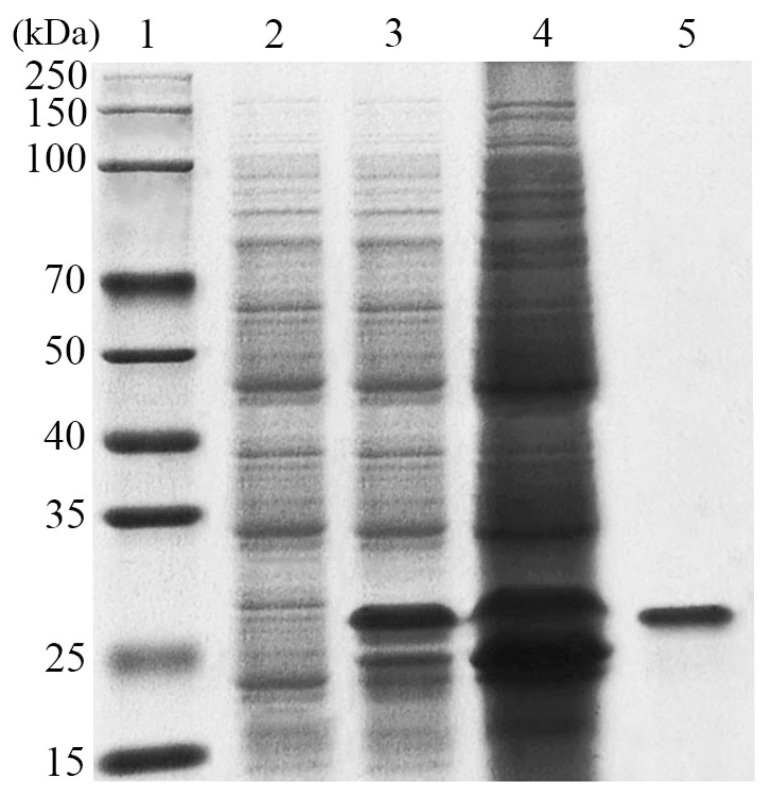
SDS-PAGE analysis of the purified recombinant *Le*Cho1 protein. Lane 1, standard molecular mass marker; lane 2, the supernatant of *E. coli* cells harboring pET29a (empty vector control) after ultrasonication; lane 3, the supernatant of *E. coli* cells harboring pET29a-*LeCHO1* (the recombinant plasmid) after ultrasonication; lane 4, the precipitate of *E. coli* cells harboring pET29a-*LeCHO1* after ultrasonication and centrifugation; lane 5, the purified *Le*Cho1 protein.
